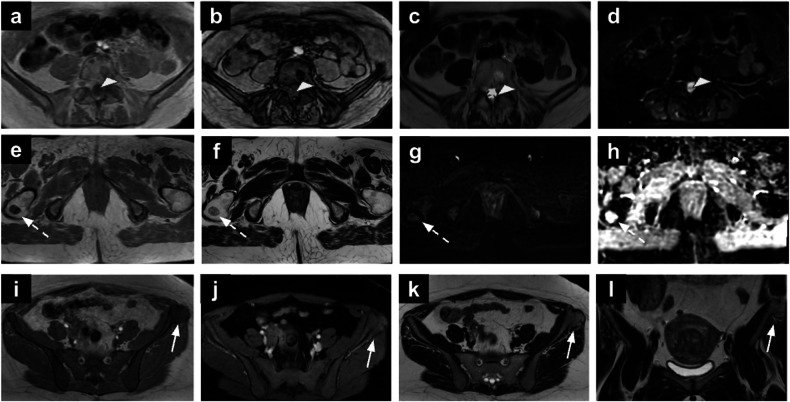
Incidental bone marrow lesions: haemangioma (a–d), enchondroma (e–h) and osteochondroma (i–l). Pelvic MRI (a–d) for cervical cancer staging in a 61-year-old patient showing a haemangioma in the L5 vertebral body. There is intralesional fat, depicted as signal drop between in-phase (a) and out-of-phase (b) images; the lesion (arrowheads) shows high SI on T2-WI (c) and moderate SI high *b*-value DWI (d). Incidental right femoral enchondroma (dotted arrows) in a 64-year-old patient: the lesion has well-defined borders, low SI on T1-WI (e) and intermediate-to-high SI on T2-WI (f), without significant diffusion restriction (g, h). Incidental left iliac osteochondroma (arrows) in a 48-year-old patient undergoing MRI for myometrial mass characterisation (i–l). The osteochondroma is characterised by the normally appearing bone marrow, continuous with the medullary cavity of the left iliac bone. SI, signal intensity; WI, weighted images
